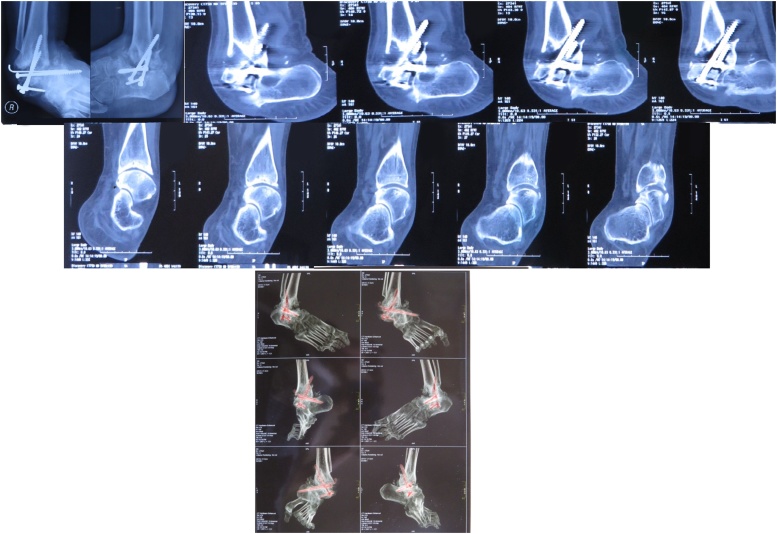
Computed Tomography (CT) Scan examination of the right foot of the first case. Destruction of tibia-fibula distal bone, talus, calcaneus and tarsal bone was observed. Hyperthrophy of metatarsal 1–5, pin on tibia with penetration soft tissue, narrowing of ankle joint, soft tissue oedema which consistent with a charcot arthropathy of right ankle-foot with pin penetration soft tissue.
